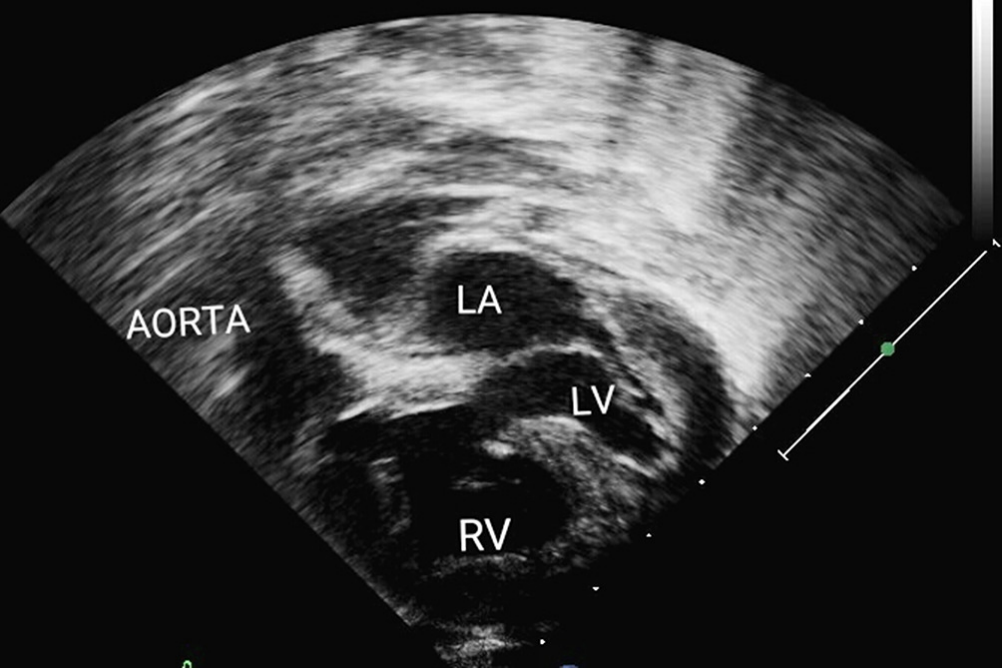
Transthoracic two-dimensional echocardiography showing the ventricular septal defect, aortic annulus and expected course of the baffle. LA, left atrium; LV, left ventricle; RV, right ventricle.
